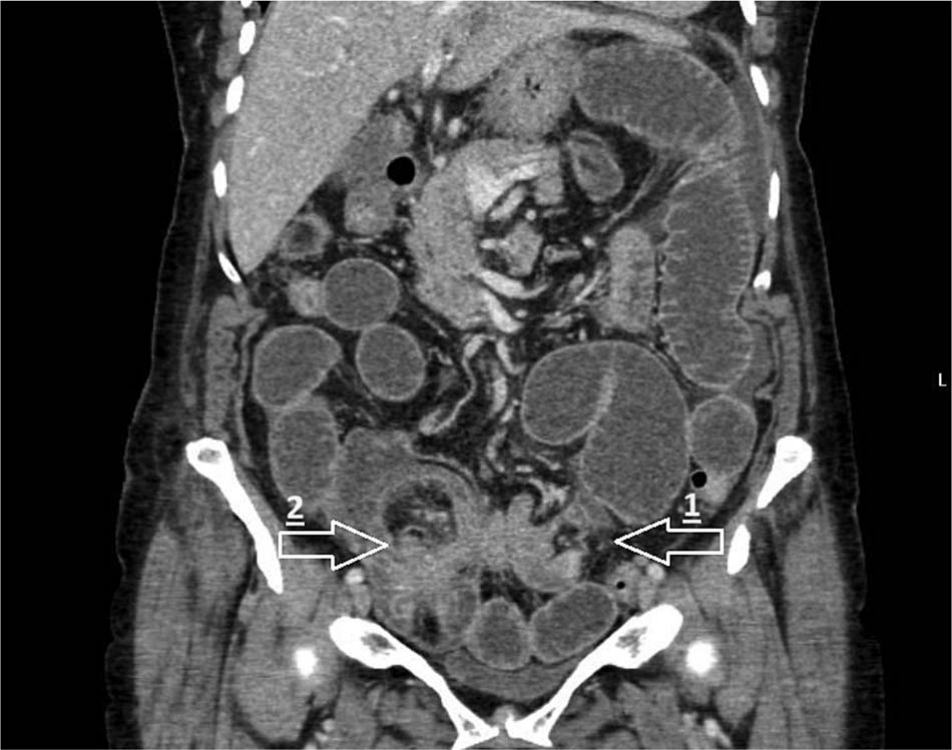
CT coronal image demonstrating small bowel obstruction secondary to ileo-colic intussusception with large segment of small bowel invaginating into caecum. Arrows mark the following: 1—transition point of bowel obstruction, 2—ileo-caecal intussusception ‘lead point’.
